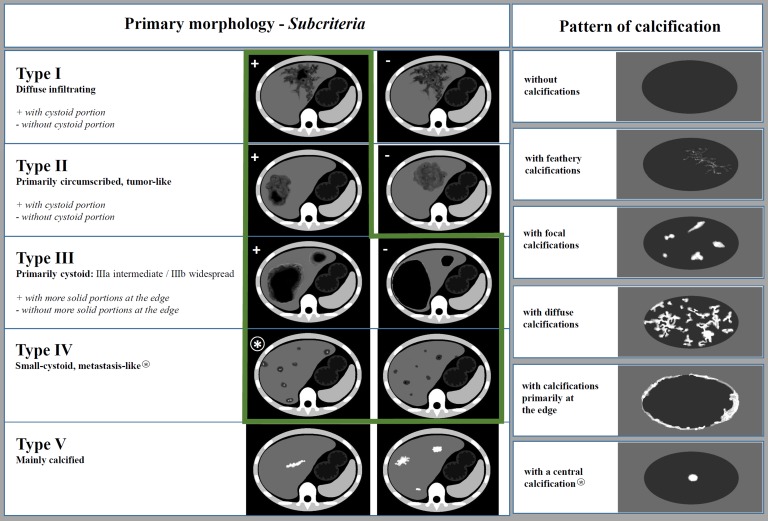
Overview of the EMUC-CT after Graeter et al. Left: Primary morphological types I–V and their subcriteria (applicable to types I, II and III). Right: Patterns of calcification. (EMUC-CT = *Echinococcus multilocularis* Ulm Classification for Computed Tomography). The two columns of the classification are primarily considered separately and can then in principal be freely combined. There are two exceptions: The pattern of calcification “with a central calcification*” can only occur with primary morphology type IV* and primary morphology type V is not further characterised by a pattern of calcification. Lesions of types I and II with cystoid portions as well as types III and IV were evaluated (marked with a green frame).
